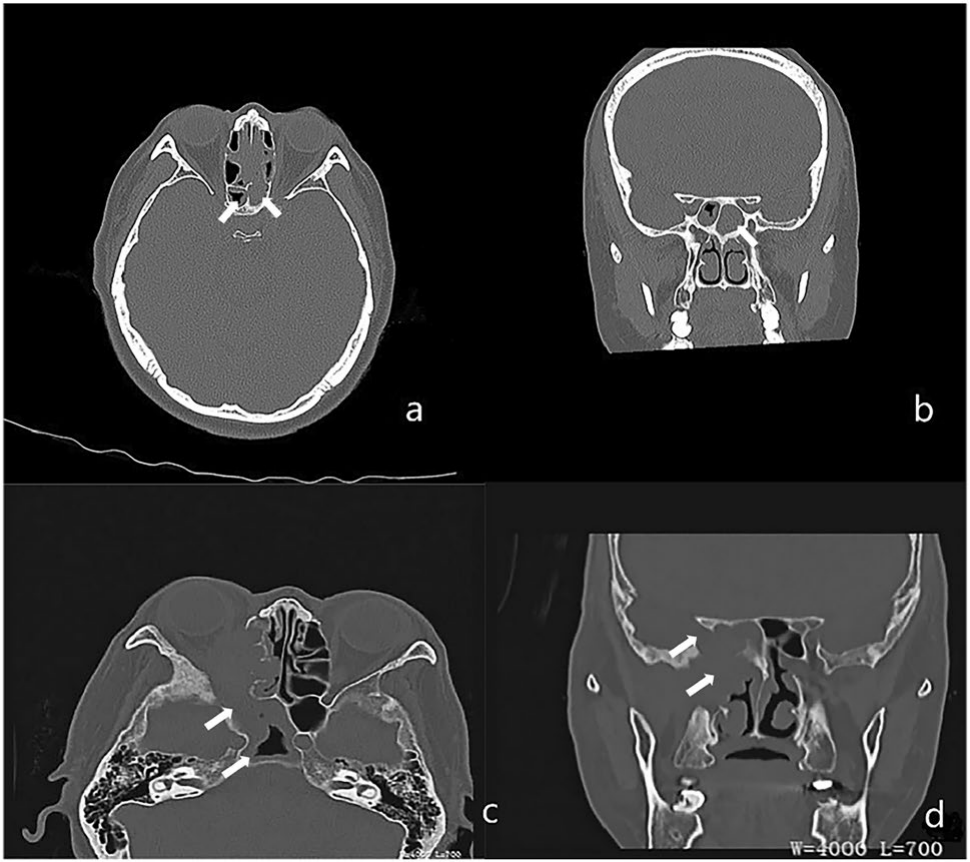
Computed tomography (CT) findings of fungal sphenoid sinusitis (FSS). (a, b) show complete opacity of the left sphenoid sinus with calcification (white arrow), part opacity of the right sphenoid sinus in noninvasive FSS (white arrow). (c, d) show complete opacity of the right sphenoid sinus with surrounding bone destruction (white arrow) in invasive FSS.
